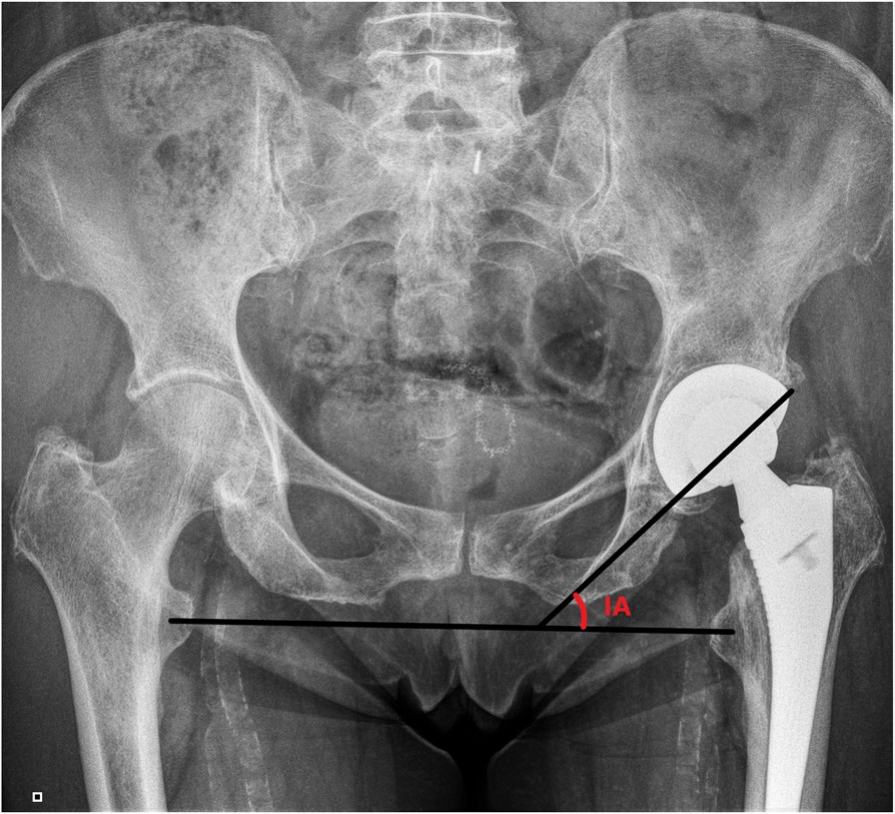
IA measurement. A case of THA with posteroanterior X-ray image shows the measurement of IA. See text for the description of this angle
